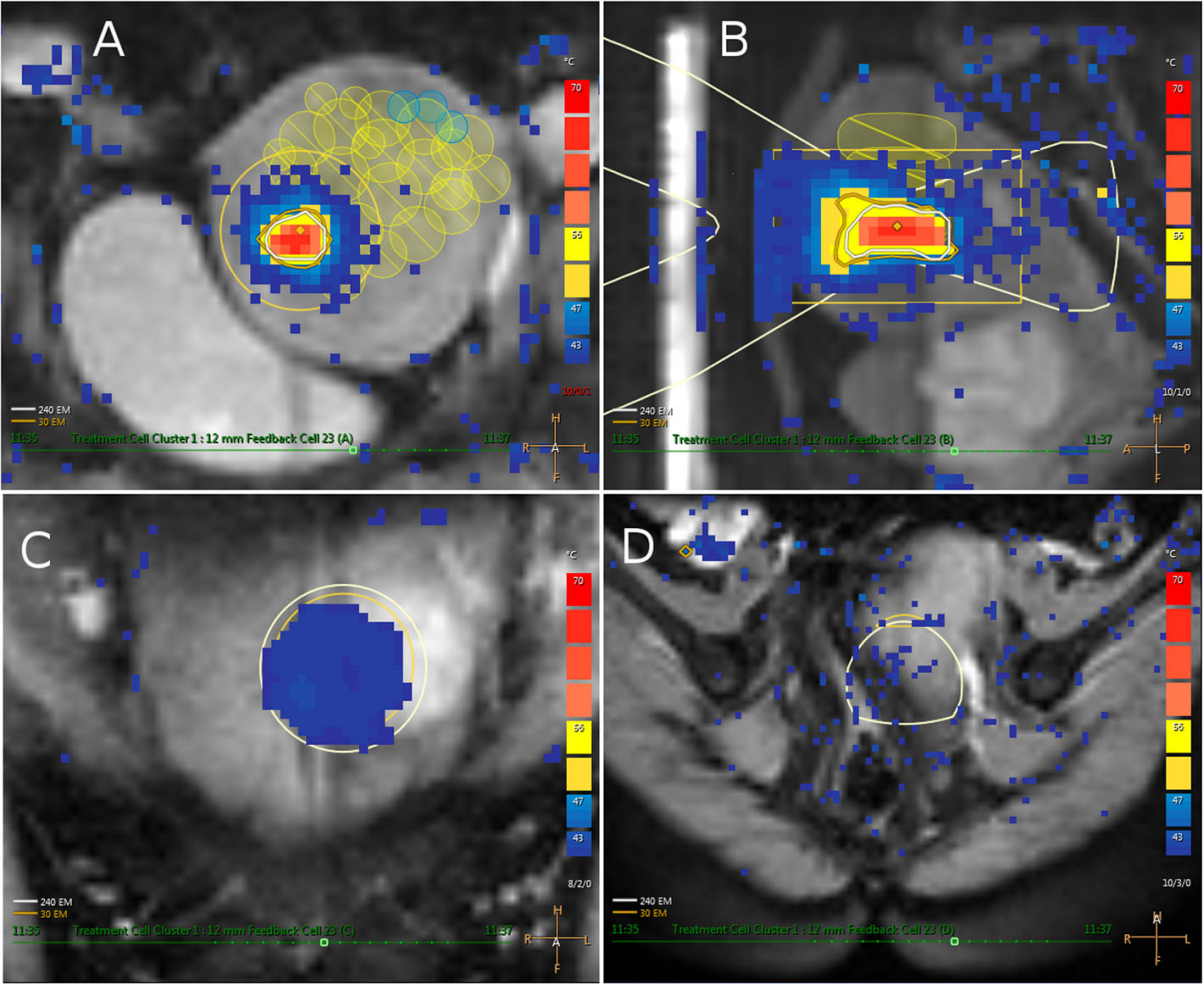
Multiplane MR thermometric images acquired during sonication with a 12-mm treatment cell (frequency, 1.2 MHz; acoustic power, 190 W) with visualization of the ultrasound focus in a coronal view, b sagittal view, and c coronal view from the near field and d oblique view from the far field
